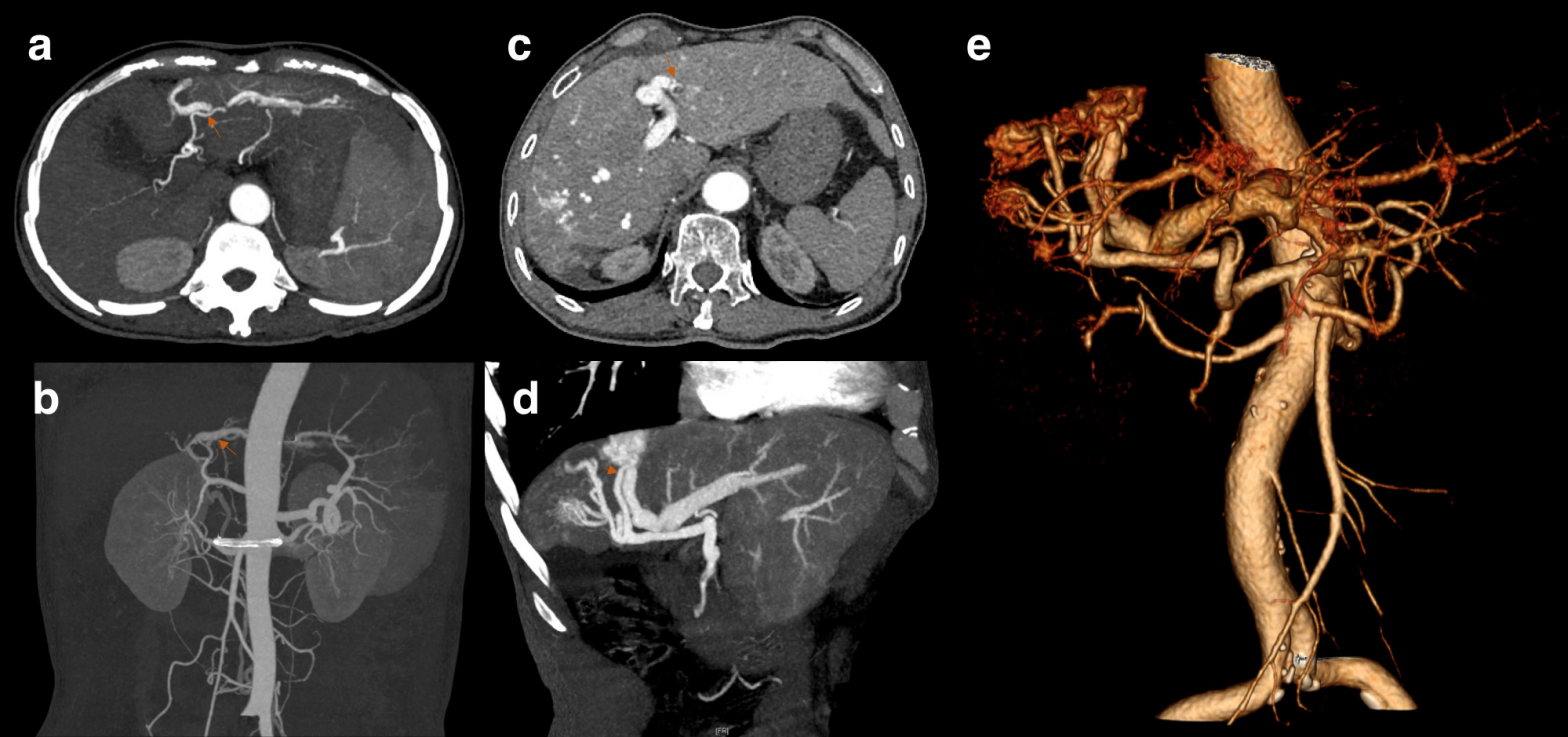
Figure a and b are from the same patient and demonstrate the mild type of IAPF a. The arterial phase of dynamic CT shows early enhancement of transient, peripheral, small hepatic vein branches. b. Maximal intensity projection (MIP) image of the portal trunk in the arterial phase shows the mild type of fistula. Figure c, d, and e are from the same patient and demonstrate the severe type of IAPF. c. Multiple fistulas developed in both central and peripheral areas. d. A large fistula was seen in the MIP image. e. Volume-rendered images show multiple fistulas in a severe type of IAPF. (Red arrows for concomitant hepatic arteries).
